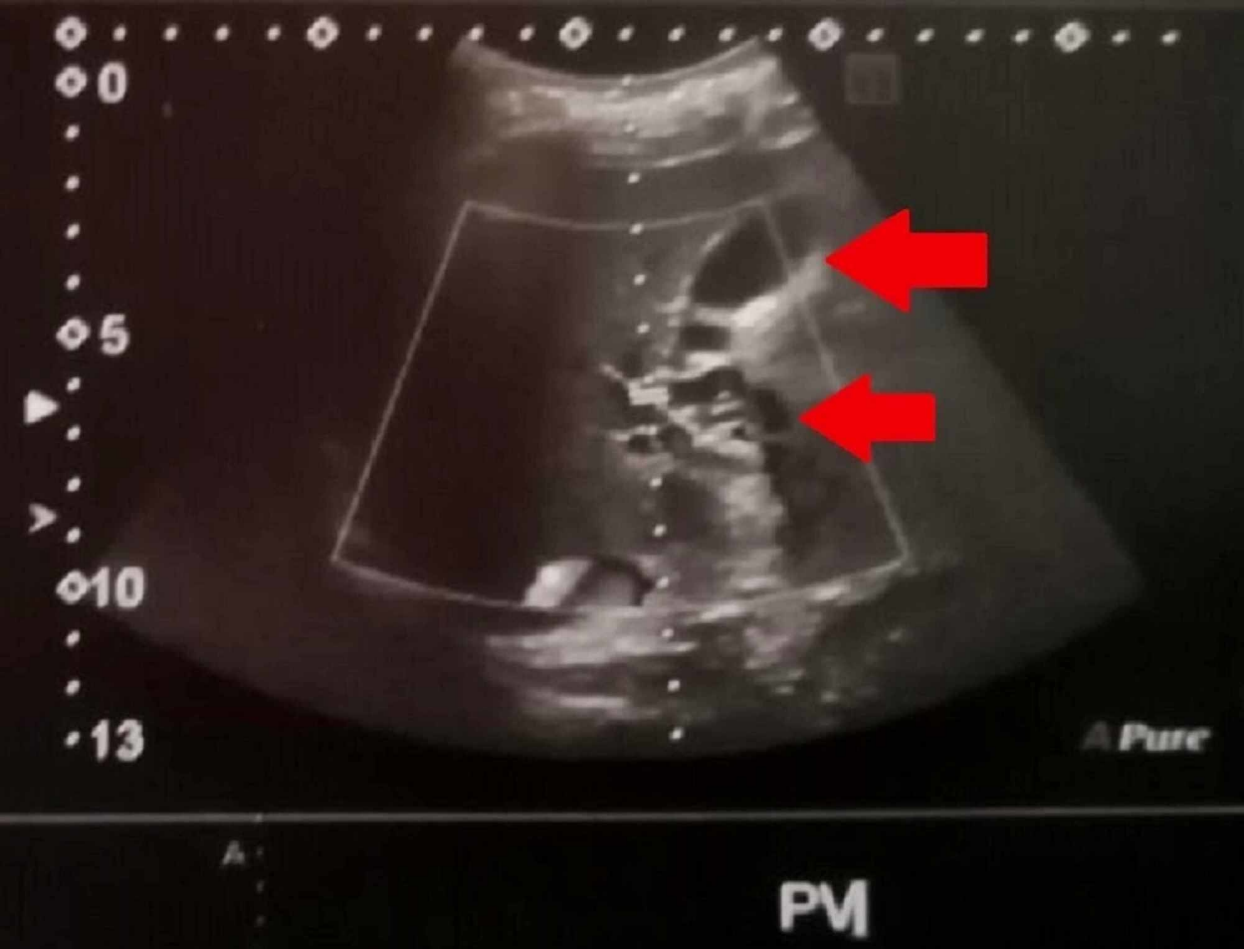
Doppler US showing CTPV (red arrows) US, ultrasound; CTPV, cavernous transformation of the portal vein
